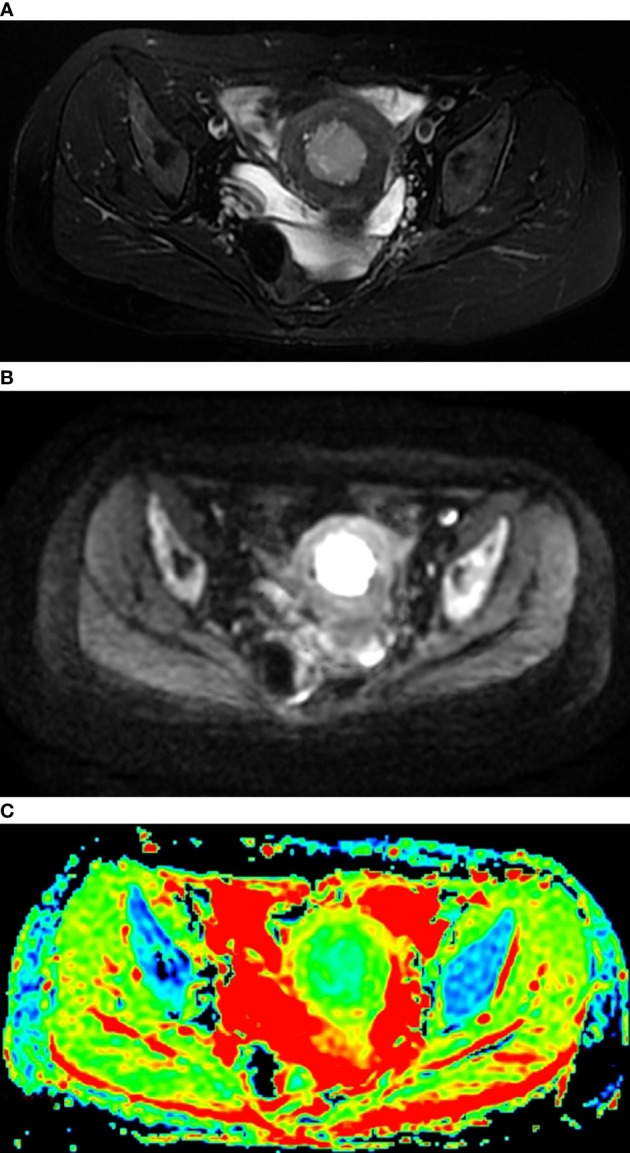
T2WI (A), DWI (B) and DW-ADC image (C) of EC.
